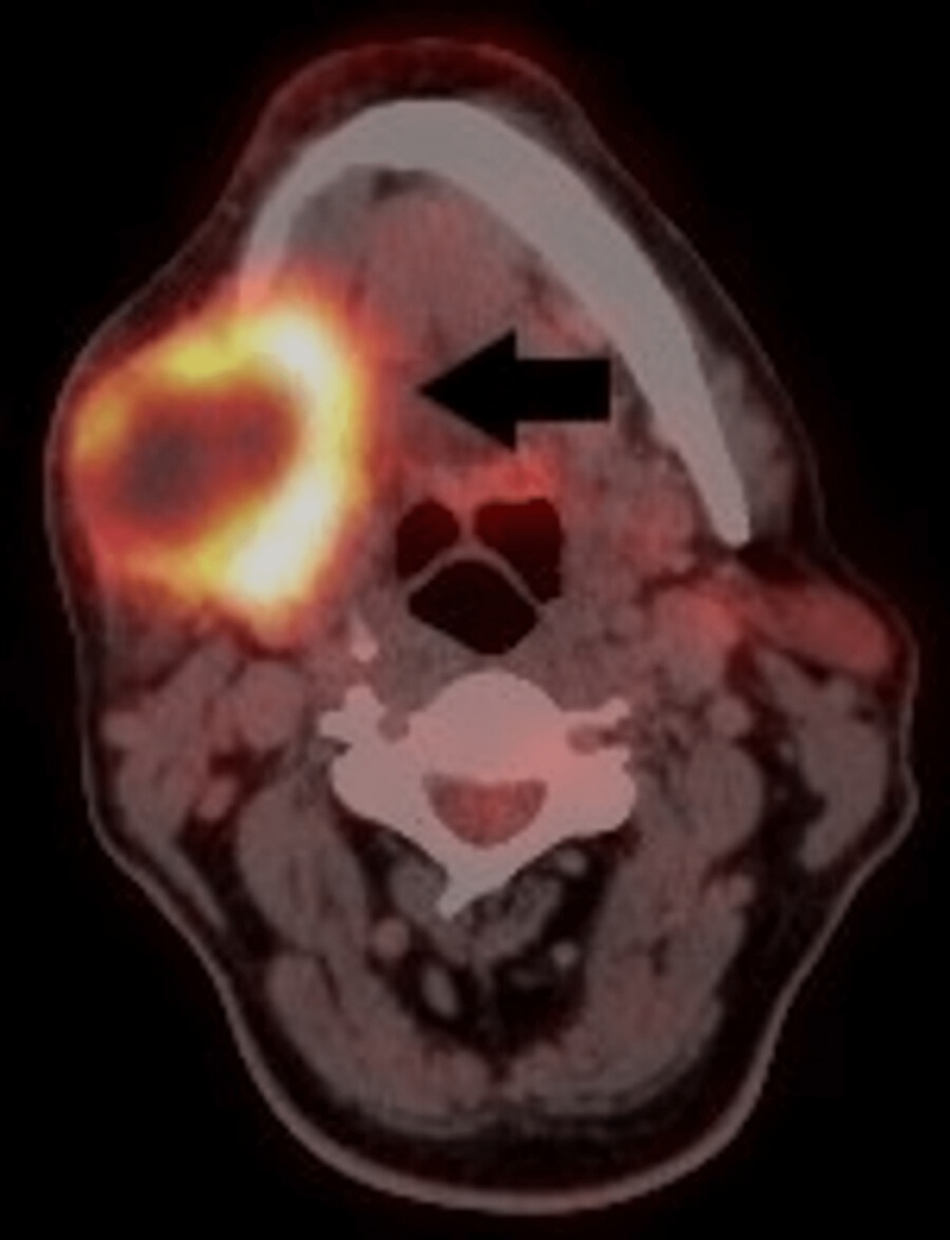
An 18F-FDG PET CT scan showing involvement of the submandibular salivary gland.
